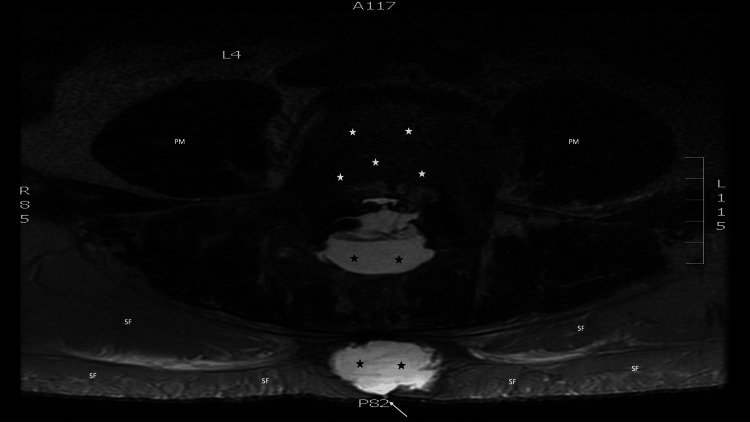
Axial view of magnetic resonance imaging showing the cerebrospinal fluid leak Twelve days after surgery, a magnetic resonance imaging of the lumber back of a 58-year-old man was performed on a 1.5 Tesla scanner, with axial T1-weighted and T2-weighted imaging. The axial view, at the level of the fourth lumbar vertebrae, demonstrates the vertebral body (white stars) and bilateral psoas muscles (labeled PM). Subcutaneous fat (labeled SF) can be seen. In addition, two collections of cerebrospinal fluid (black stars) are present in the soft tissue of the back; a tract (white arrow), extends from one of the soft tissue collections of cerebrospinal fluid to the surface of the skin surface.
